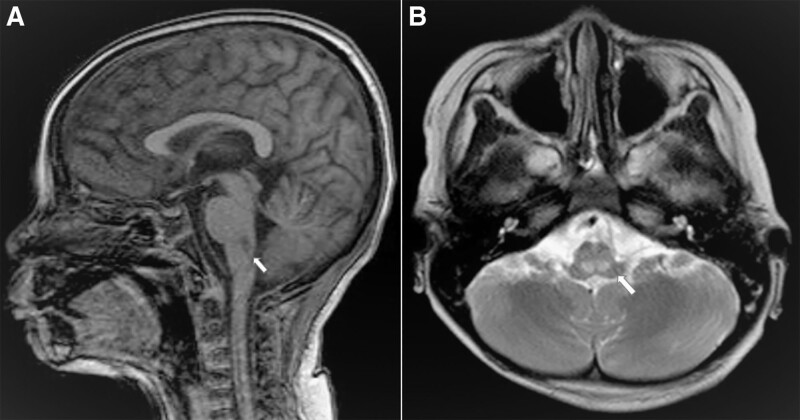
Magnetic resonance imaging (MRI) revealed small nodular lesions in the posterior medulla oblongata bilaterally, as indicated by the white arrows in the sagittal plane (A) and axial views (B).
